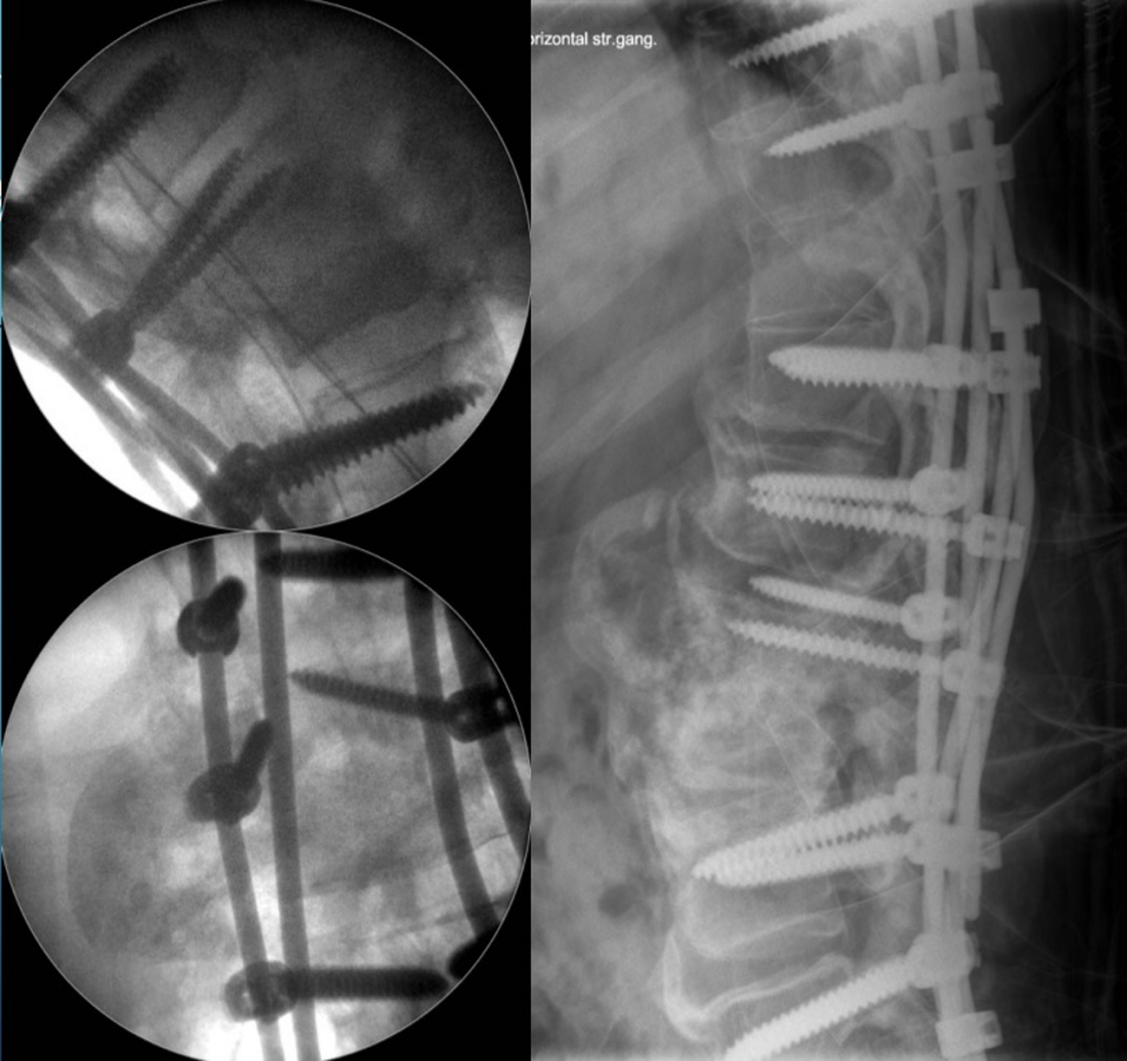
Intra-operative fluoroscopy image showing final fixation with four rod construct and immediate post operative radiograph showing good 3D correction of the deformity with stable fixation.
